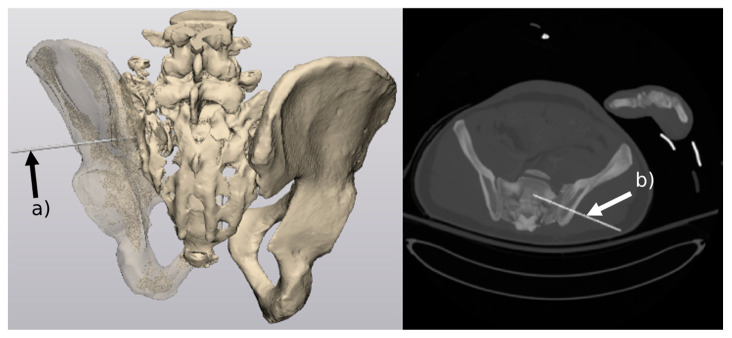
(a) placement of the guide in 3-matic, (b) maximum intensity projection (MIP) of 30 CT slices displaying the location of the guide and its insertion depth.
